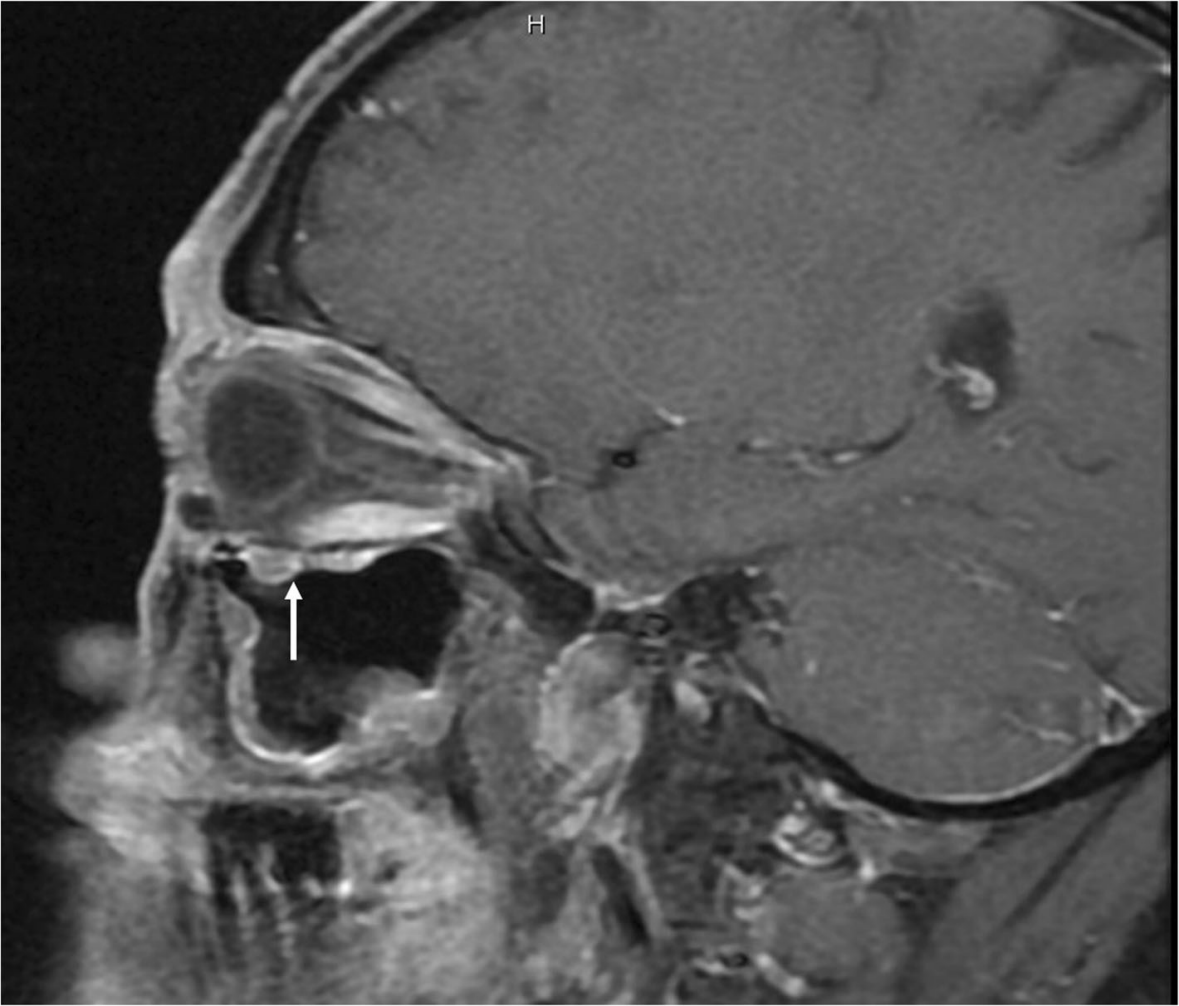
Optic nerve sheath enhancement in patients accompanied with IONE. Sagittal contrast enhanced T1-weighted MRI shows enhancement of optic nerve sheath with IONE (white arrowhead)
